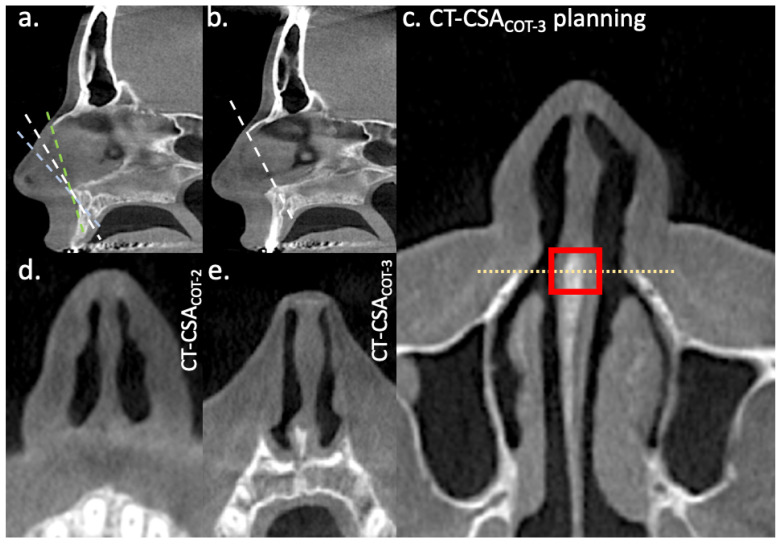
Identification of the planes of Cottle-area-2 and Cottle-area-3 at the sagittal plane of the septum. The selected oblique planes for (a) Cottle-area-2 and (b) Cottle-area-3 are indicated by the white dashed line. The blue and green dashed line in (a) indicates the oblique planes of the CT-CSA titled about 30° and 60° to the nasal floor, respectively, examined in another study [9]. The cross-sectional areas of (d) CT-CSA_COT-2_ and (e) CT-CSA_COT-3_ correspond to (a) and (b), respectively. The head of the inferior turbinate is visible in the plane of Cottle-area-3 (e). Planning of the CT-CSA_COT-3_ is depicted in (c). The yellow pointed line indicates the level of the anterior borders of the ascending processes of the maxilla, and the red rectangle indicates where the plane of Cottle-area-3 starts.
